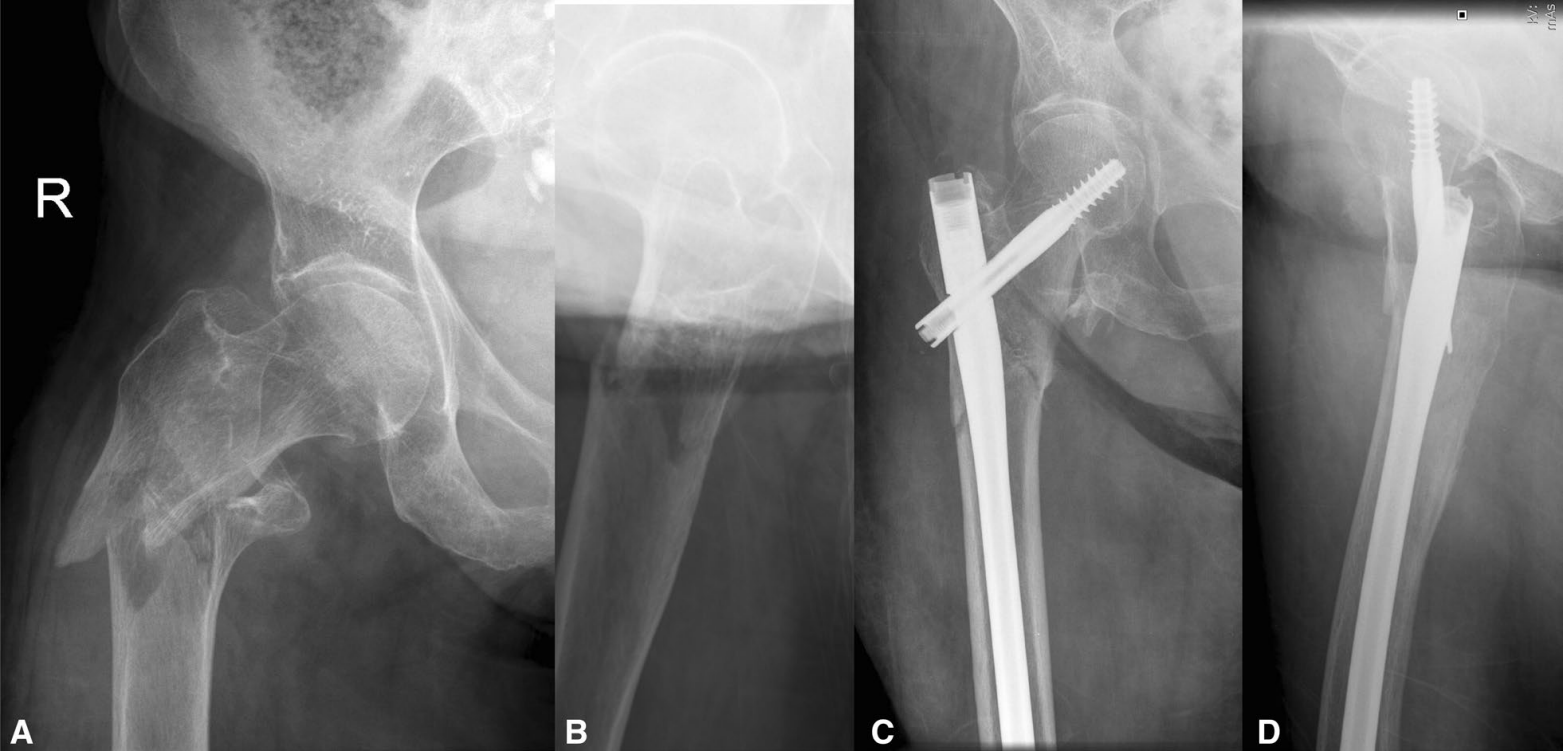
Pre-operative AP (A) and lateral (B) radiographs and post-operative AP (C) and lateral (D) radiographs of a subtrochanteric fracture managed with a Gamma nail
